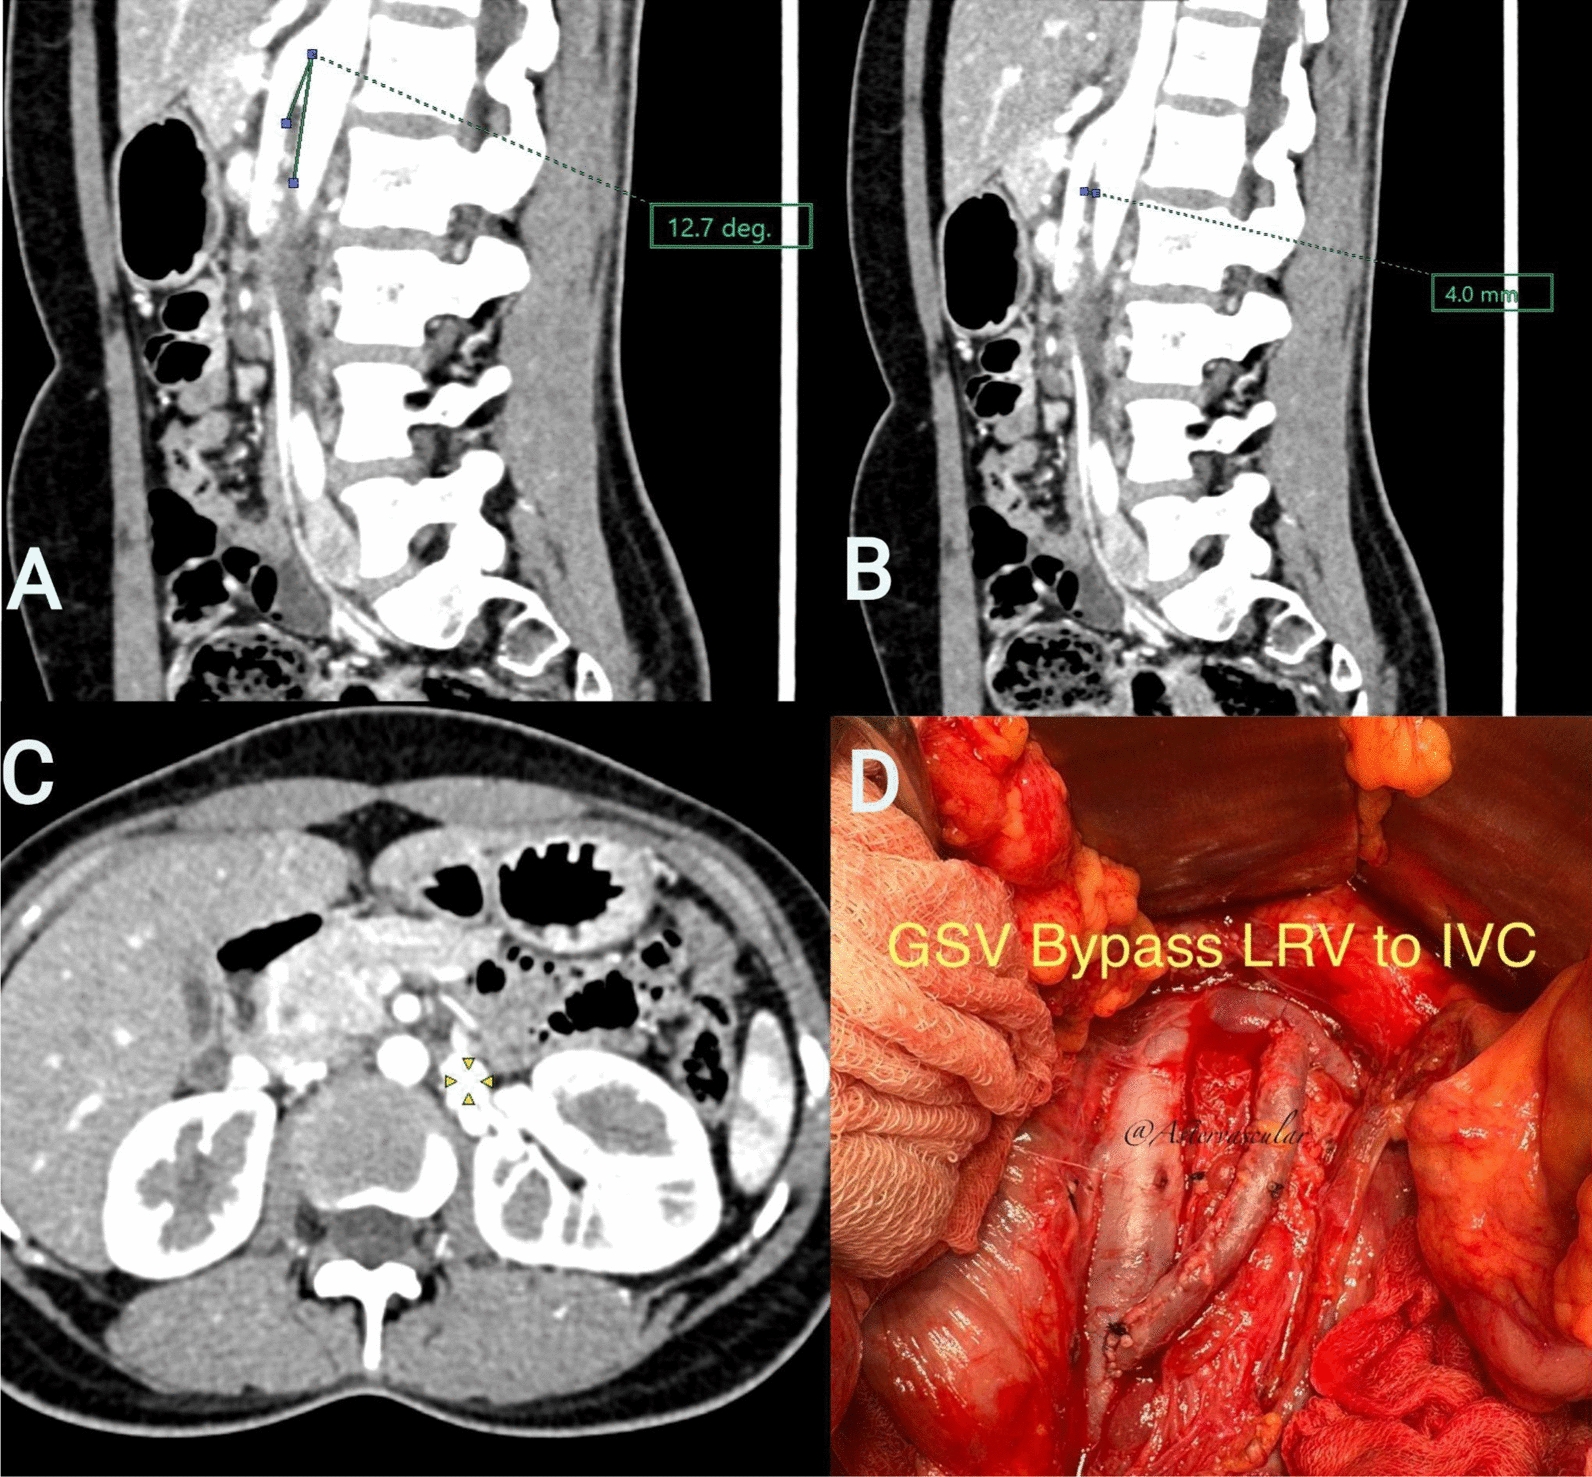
A Computed tomography angiogram sagittal section showing aortomesenteric angle of 12.7° with minimal dilatation of gonadal vein; B computed tomography angiogram sagittal section showing aortomesenteric distance of 4 mm; C computed tomography angiogram axial section showing dilated renal vein (arrows); D intraoperative image showing left renal vein bypass grafting was done using the left greater saphenous vein
